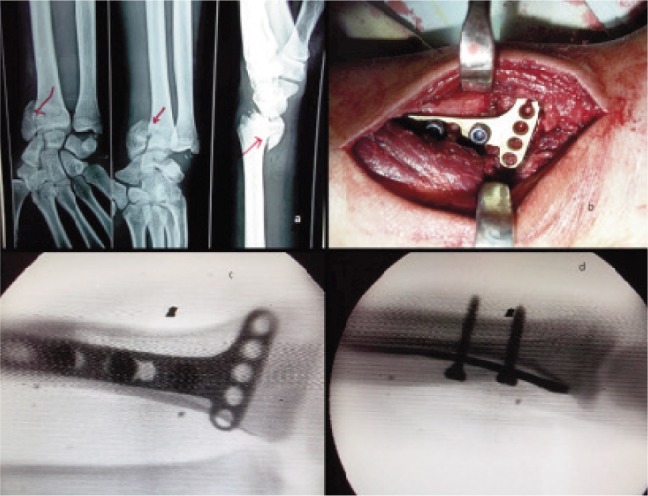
a) preoperative radiographs showing intra articular distal end radius fracture with open physis, b) intra operative radiograph showing screws only proximal to fracture site, c) & d) image intensifier views of reduced fracture using volar buttress plate.
